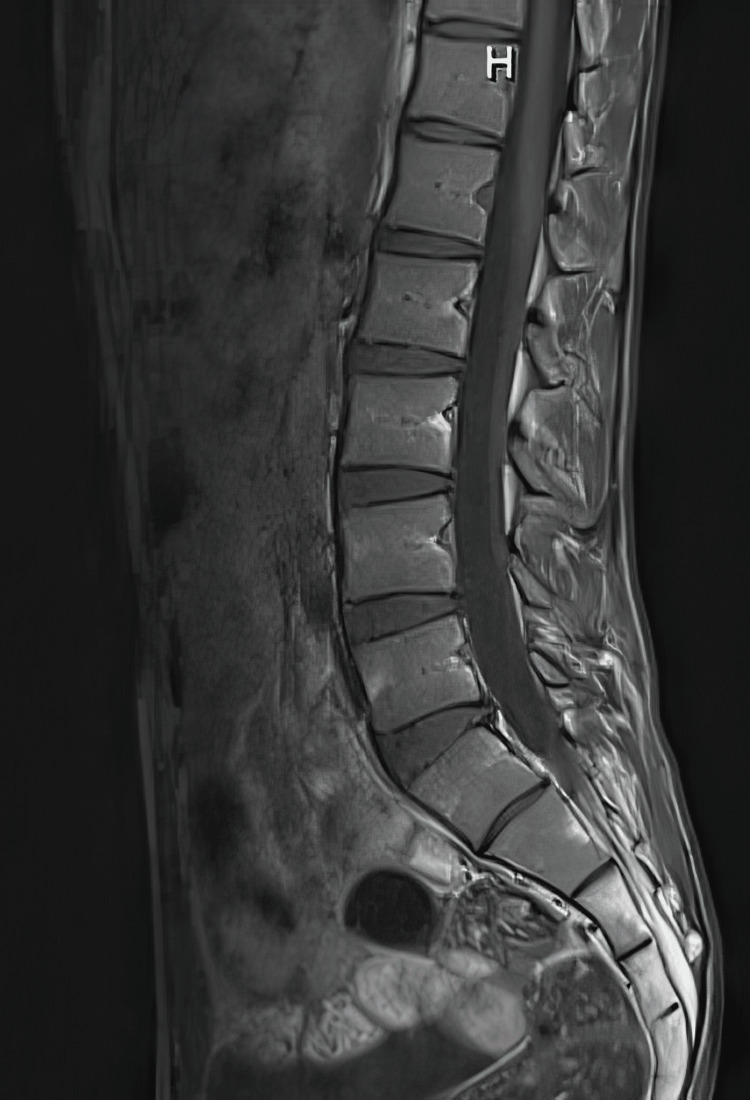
Normal MRI lumbar spine, T1 weighted image
